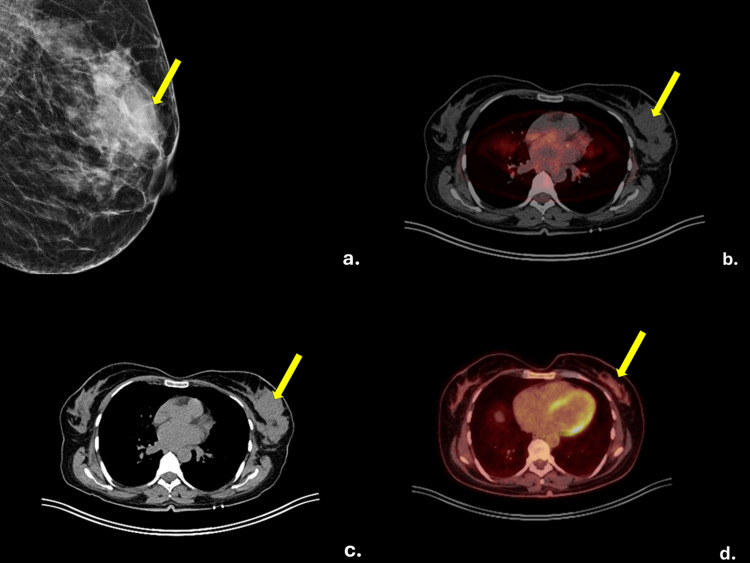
Diagnostic images a. Mammography showing dense breast tissue ACR-type C b. Axial fused positron emission tomography–computed tomography (PET-CT) (pre-treatment) demonstrating intense F-18 fluorodeoxyglucose (FDG) uptake in the left breast mass and ipsilateral axillary lymph nodes, consistent with metabolically active malignancy. c. An axial CT image (pre-treatment) showing a dense soft tissue mass in the UOQ of the left breast, with associated left axillary lymphadenopathy. d. Axial fused PET-CT (midtreatment-4 cycles RCHOP) demonstrating complete metabolic response with no residual FDG uptake in the left breast or axilla. ACR: American College of Rheumatology, RCHOP: Rituximab, Cyclosphamide, Hydroxydaunorubicin, Vincristine (Oncovin), Prednisone.
